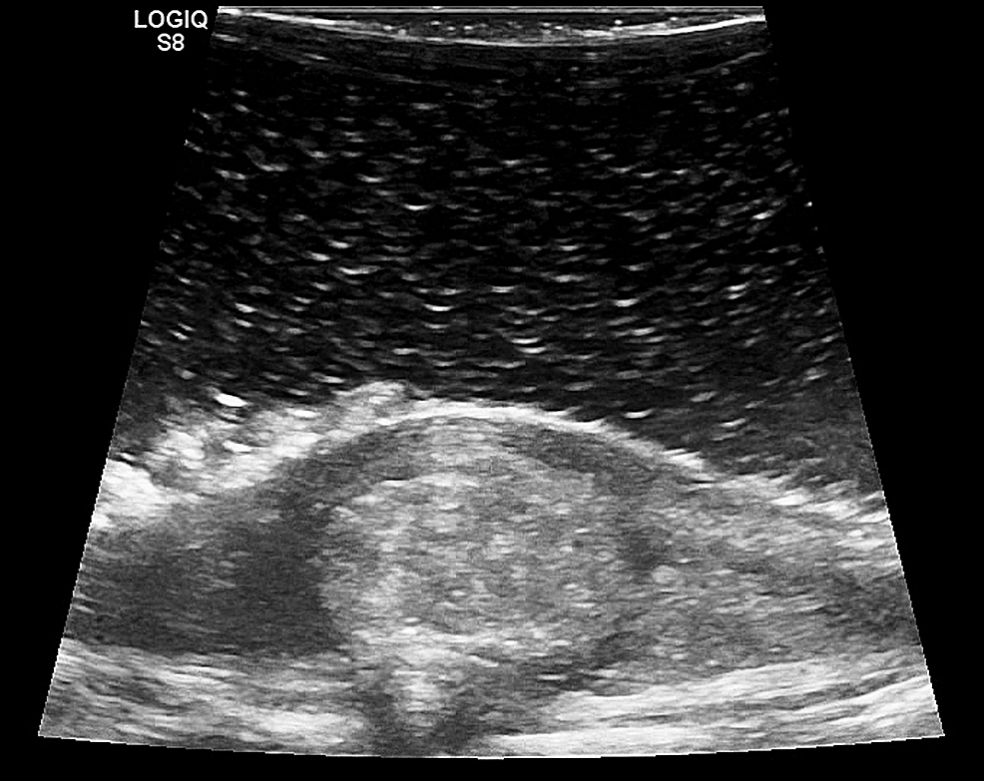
Intraoperative ultrasound findings. In the sagittal plane, the lesion was located at the L3-L4 level, but there was no continuity with the disc. Dura continuity was identified between the lesion and the ventral low echoic region.
